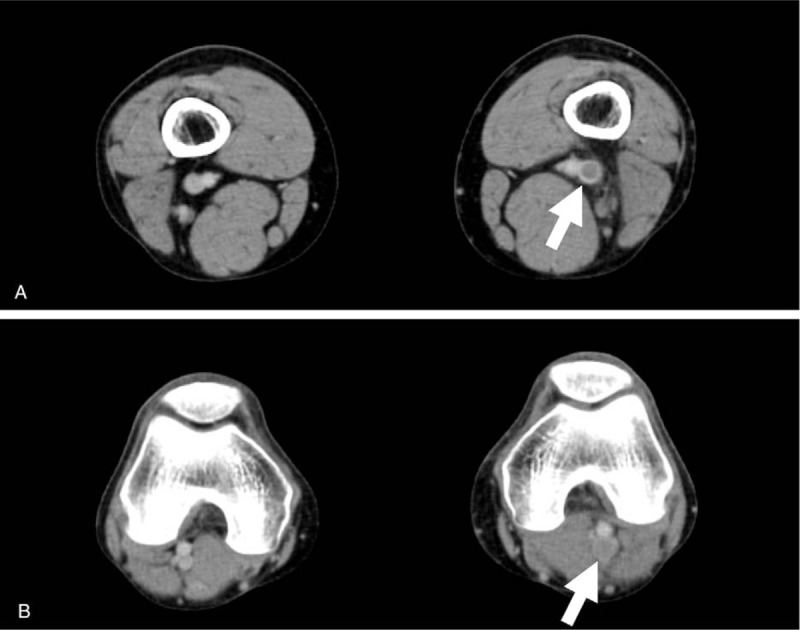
Contrast enhanced computed tomography on admission showing a thrombus from left femoral vein (A) to the left popliteal vein (B) (arrows).
